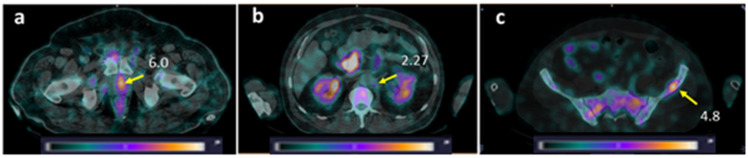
SPECT/CT images of PCa patients 2 h after injection of [^99m^Tc]Tc-DB8. The foci of increased [^99m^Tc]Tc-DB8 uptake (yellow arrow, SUV_max_) are visualized (a) in the prostate (Patient 16); (b) in the paraaortic lymph node on the left (Patient 10); and (c) in the left ilium (Patient 10). A linear relative scale (normalized at the maximum activity in the image SUV_max_ from 0 to 5.0) is applied.
